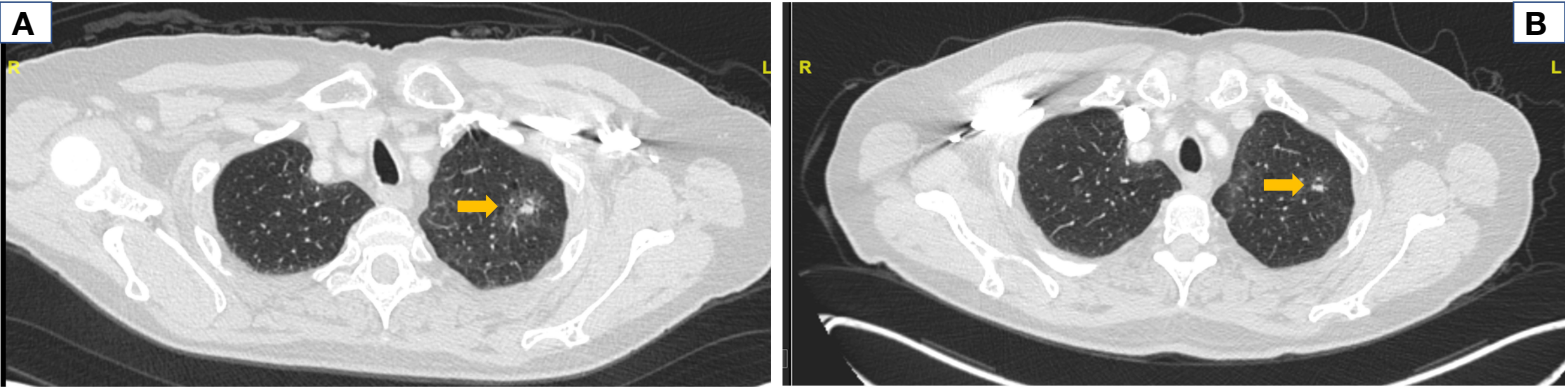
Radiologic response to neoadjuvant selpercatinib. Computed tomography (CT) imaging of chest before and after neoadjuvant selpercatinib treatment is shown. (A) A baseline CT of the chest before selpercatinib initiation revealed a left lung mass measuring 3.1 cm (arrow). (B) A follow-up CT of the chest after 8 weeks of selpercatinib showed disease regression to 2.9 cm in the same mass (arrow). This was classified as stable disease by RECIST v1.1 per investigator assessment. RECIST, Response Evaluation Criteria in Solid Tumors.
